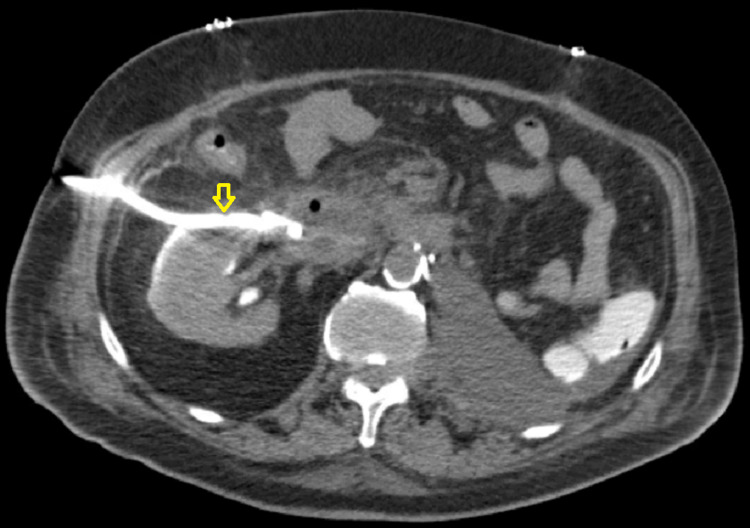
Repeat CT abdomen showing percutaneous drainage tube placement (yellow arrow)
